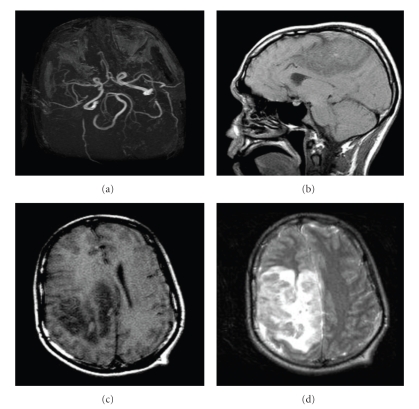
(a) MRI angiogram showing patent cerebral arteries. ((b), (c), (d)) Sagittal and transverse MRI views, T1 and T2 weighed, depict a large right hemorrhagic lesion with prominent edema and midline shift.
